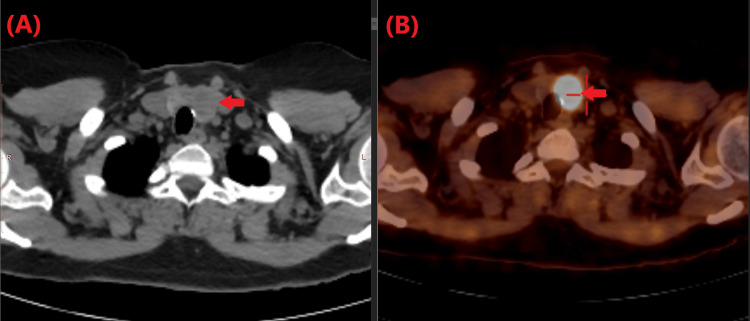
CT scan (A)/ PET scan (B) at the level of thyroid gland showing 1.8 cm left thyroid nodule (arrows) with significantly increased F-18 FDG uptake. CT,  computerized tomography; PET, positron emission tomography; FDG, fluorodeoxyglucose
